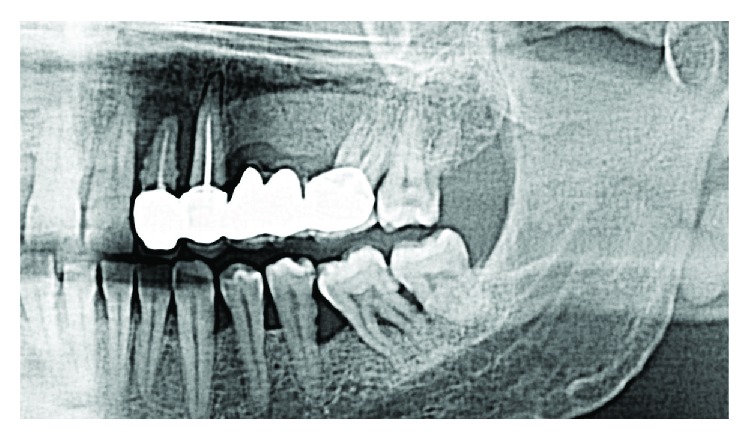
Orthopantomography previous to dental extractions.
